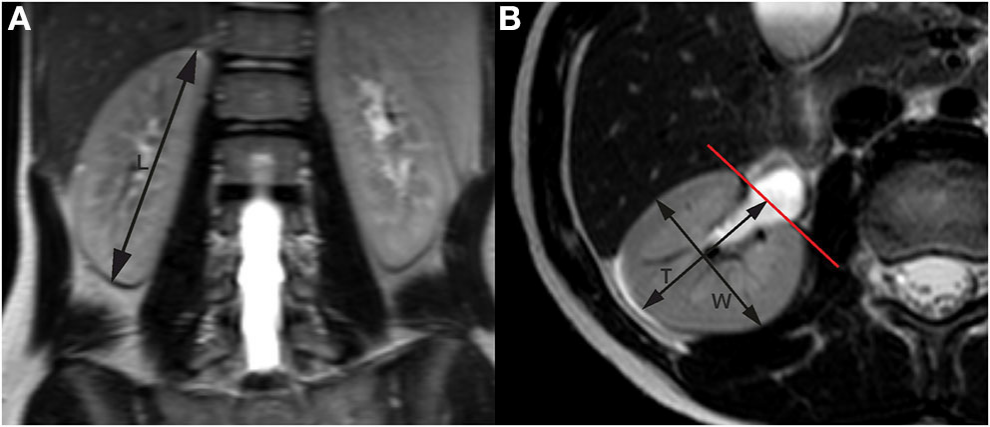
Renal measurements by MRI. (A) Longitudinal measurements in coronal plane; (B) transverse section at the level of the renal hilum. MRI, magnetic resonance imaging.
